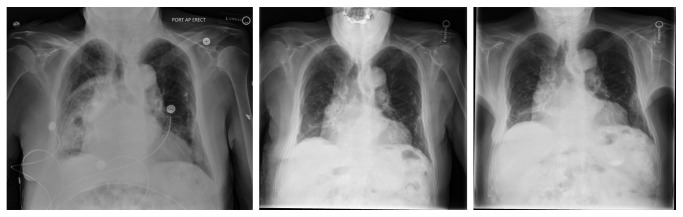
Chest X-rays from admission and at 1-month and 3-month follow-ups.
